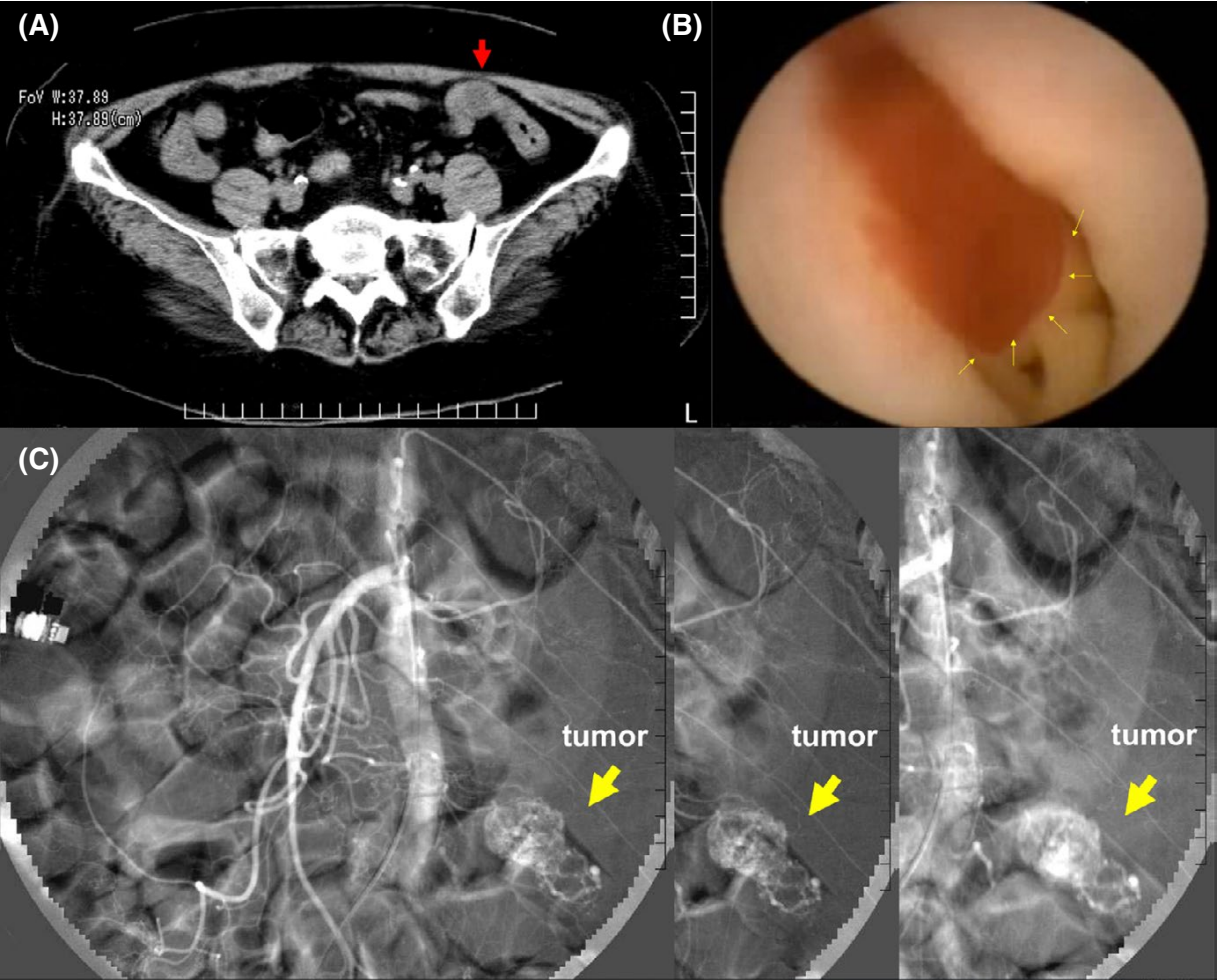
A, Abdominal computed tomography scan. CT scan of the abdomen showing a mass lesion. B, Capsule endoscopy. Capsule endoscopy showing active bleeding from the tumor in the upper small bowel. C, Abdominal angiography. A contrast agent from the superior mesenteric artery indicating a vascular tumor in the jejunal artery branch. Embolization with coils was performed in the imaged area
